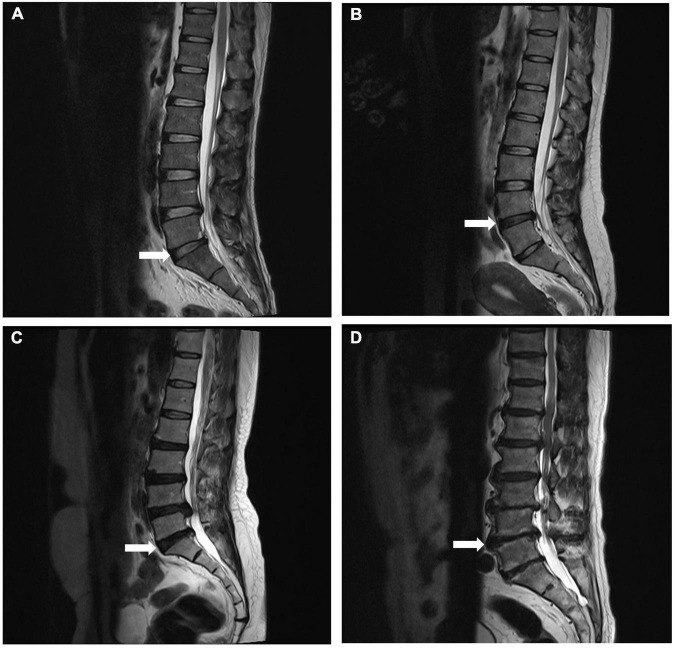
Representative MRI images used for Pfirrmann grading system of lumbar disc degeneration. Midsagittal T2-weighted views showing grade 2 (A), grade 3 (B), grade 4 (C), and grade 5 (D) degeneration of the lumbar disc.
